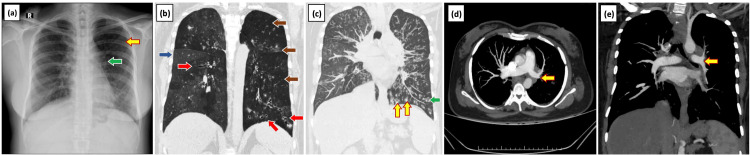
Swyer-James syndrome diagnosed in a 39-year-old female with a history of recurrent respiratory tract infection in childhood (a) Chest radiograph shows unilateral hyperlucency of the left hemi thorax (yellow arrow) with small left hilum (green arrow), reduced vascular markings in the left hemithorax, and apparent small left descending pulmonary artery. CT Coronal MinIP (b) and coronal MIP (c) reconstructed images of the chest show mosaic attenuation (blue arrow) in the right lung and bilateral bronchiectasis (red arrow) with associated mucus plugging (yellow arrow) more pronounced in the left lung. There are diffuse hypoattenuation (brown arrow) and a relatively small volume of the left lung. Small centrilobular nodules (green arrow) in both lungs suggest an active infection. Axial section CT pulmonary angiogram (d) and reconstructed coronal MIP images (e) show decreased caliber of the left main pulmonary artery (yellow arrow) and its lobar and segmental branches. MIP: Maximum intensity projection
